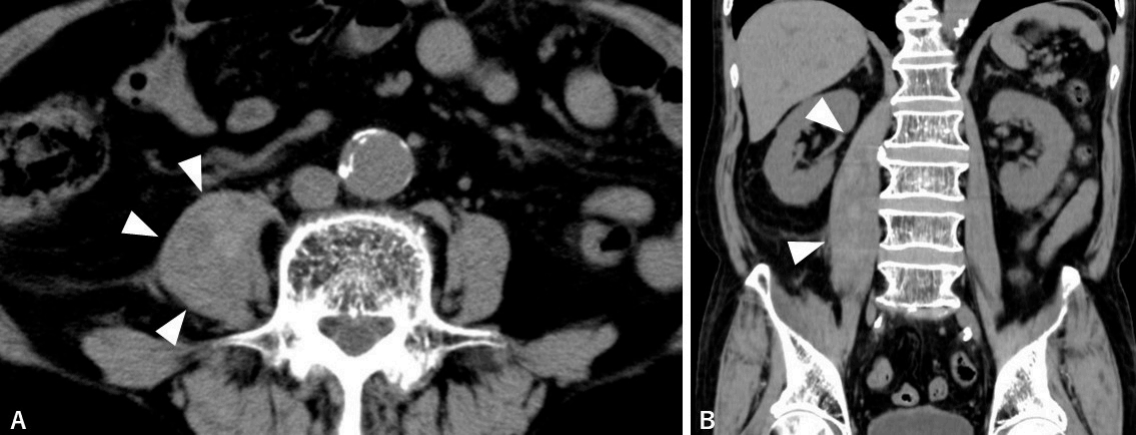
Shrunk hematoma (white arrowheads) on non-contrast CT on fourth day. (A) Horizontal view. (B) Coronal view.
